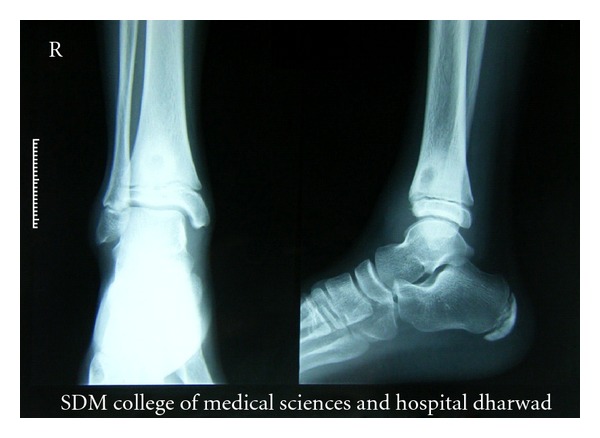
X-ray of right ankle joint showing submetaphyseal osteolytic lesion with sclerotic margin, seen in distal end of right tibia.
